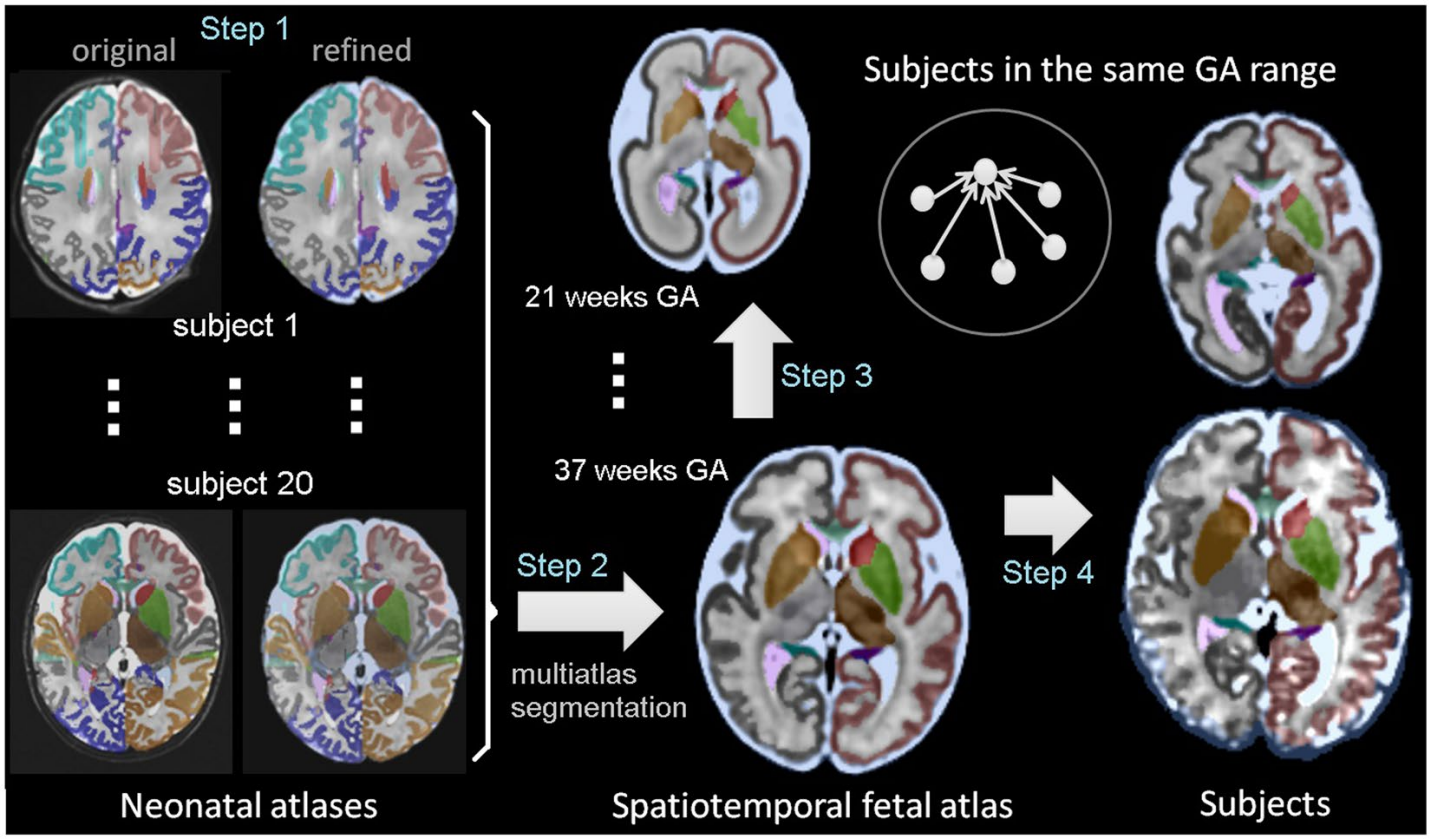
The procedure to generate atlas labels and segmentations: Step 1: labels and tissue-type segmentation of 20 neonatal ALBERTS atlases^66, 67^ were refined manually. Step 2: The segmented neonatal atlases were used to generate initial labels on the spatiotemporal fetal brain MRI atlas at higher GAs (35–37 weeks) through multiatlas segmentation using probabilistic label fusion^65^. Step 3: Fetal brain MRI labels were manually defined and propagated in iterations from the higher GAs to the lower GAs. Step 4: Atlases within one week of any query subject were used to generate an initial segmentation of that subject. The initial segmentations of all subjects within one week of the query subject were used to segment that subject.
